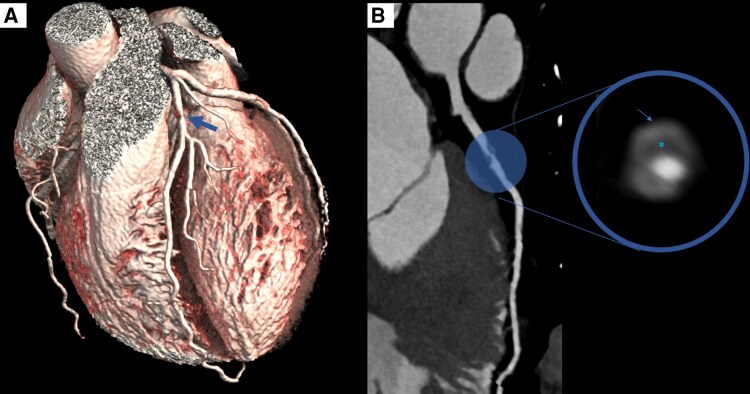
Cardiac coronary computed tomography angiography in a 47-year-old female patient with atypical chest pain and mammary tumour. Severe stenosis of the left anterior descending coronary artery is demonstrated on three-dimensional volume-rendered reconstruction (*A*, arrow) and multiplanar reconstruction (*B*). The stenosis is caused by a plaque exhibiting high risk features, with the ‘napkin-ring sign’, a low attenuation plaque (*B*, asterisk) with a hyperdense cap (*B*, arrow).
